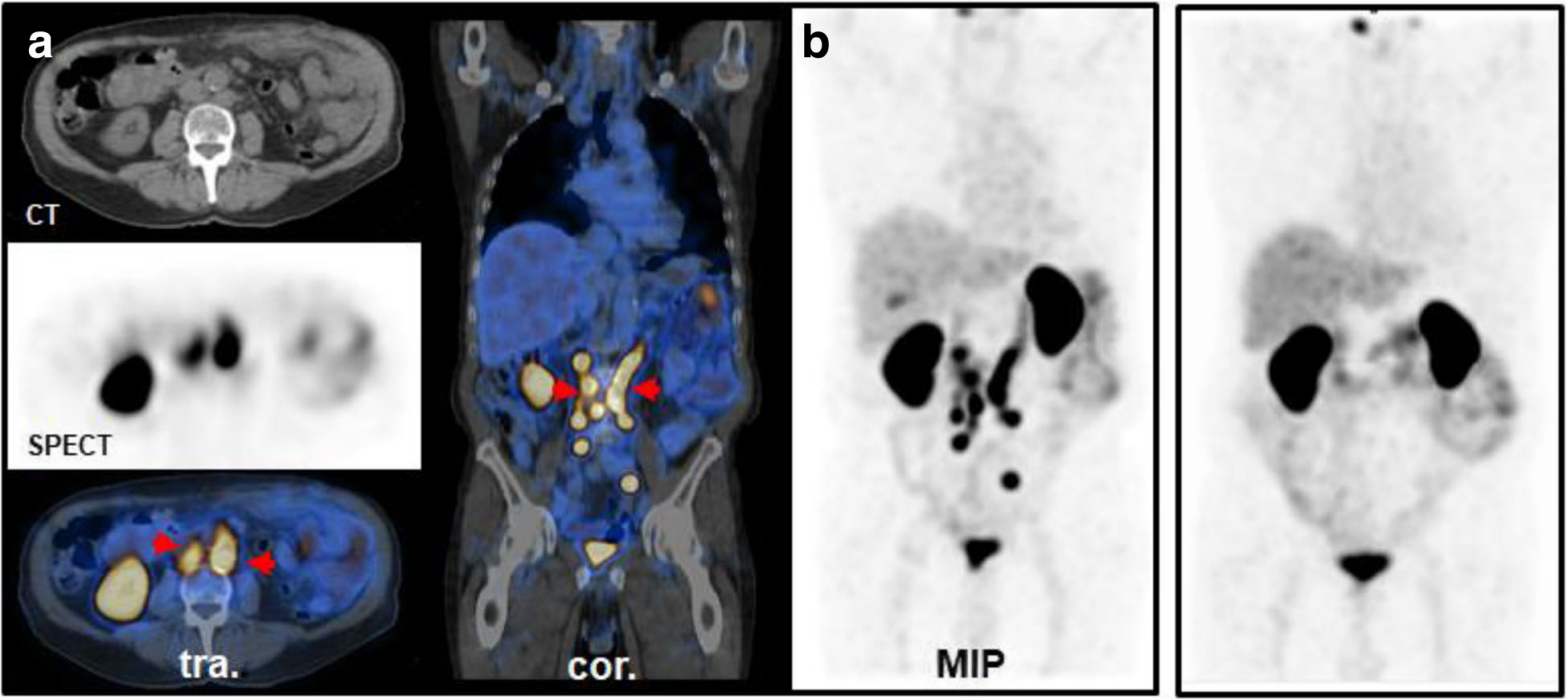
[^99m^Tc]Tc-PSMA-I&S-SPECT/CT of a 71-year-old patient with mCRPC and disease progression and severe fatigue after the second cycle of chemotherapy (docetaxel). a Pretherapeutic tumor spread in paraaortic and pelvic lymph nodes (PSA 335 ng/ml). b Restaging 3 months after 2 cycles of ^177^Lu-PSMA therapy (PSA 0.6 ng/ml). [^99m^Tc]Tc-PSMA-I&S-SPECT/CT was negative
